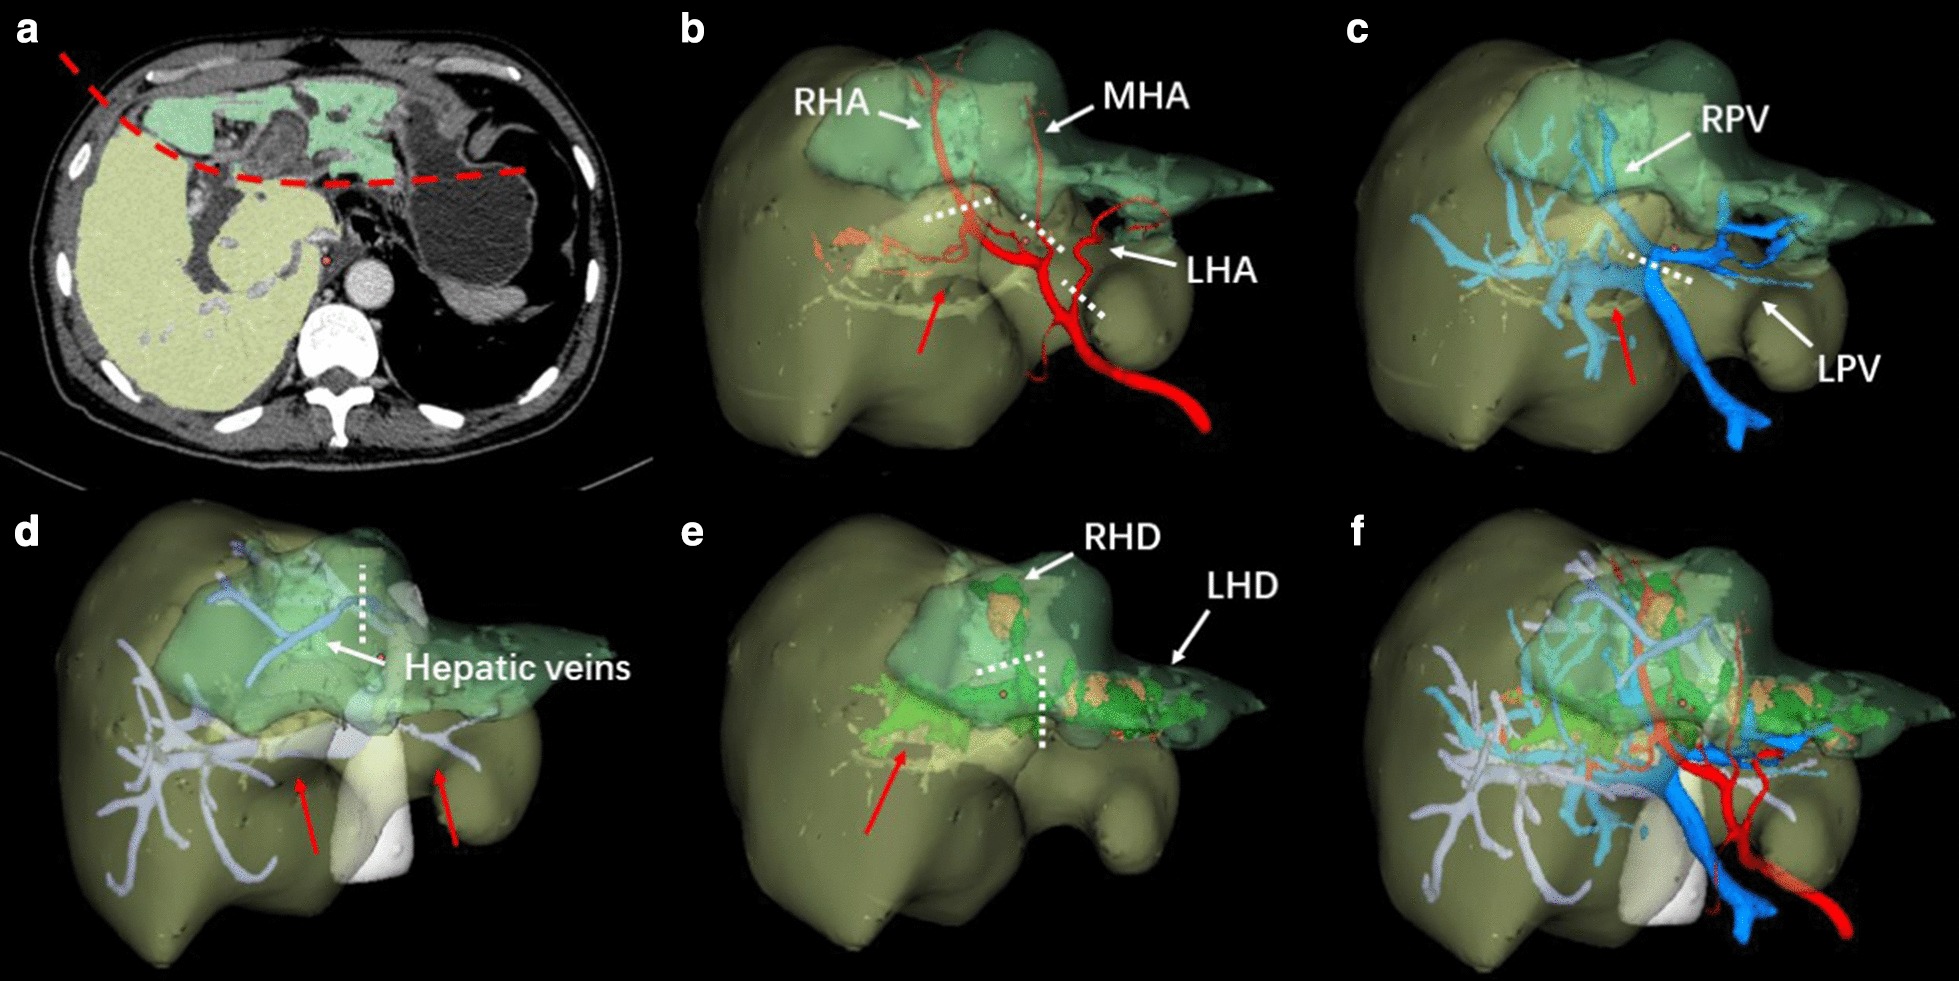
3D liver reconstruction of case 2. a Reflection of 3D reconstruction in CT image. Yellow part: hypertrophic caudate lobe; Green part: atrophic main liver; dashed red line: boundary of caudate lobe and main liver. b Reconstruction of hepatic arteries. Dashed white line: transection of hepatic arteries; Solid red arrow: hepatic artery of caudate lobe. c Reconstruction of hepatic veins. Dashed white line: transection of portal veins; Solid red arrow: portal vein of caudate lobe. d Reconstruction of hepatic veins. Dashed white line: transection of hepatic veins; Solid red arrow: short hepatic veins. e Reconstruction of bile ducts. LHD and RHD were filled with stones. Dashed white line: transection of hepatic ducts; Solid red arrow: bile duct of caudate lobe. f Configuration of the vasculatures. *LHA* left hepatic artery, *MHA* middle hepatic artery, *RHA* right hepatic artery, *LPV* left portal vein, *RPV* right portal vein, *LHD* left hepatic duct, *RHD* right hepatic duct
